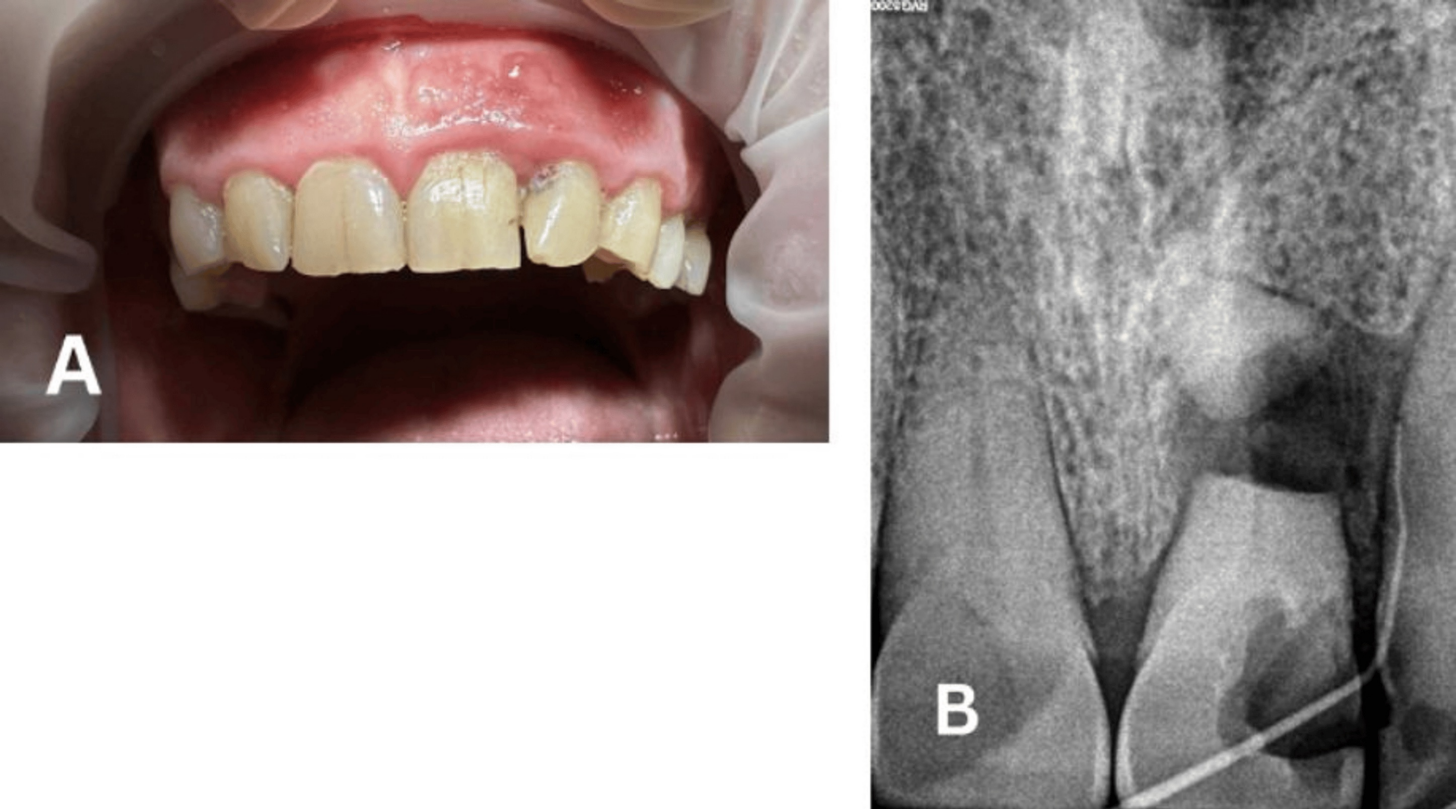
(A) Initial Clinical Picture and (B) Initial Radiograph (Fistulogram)
